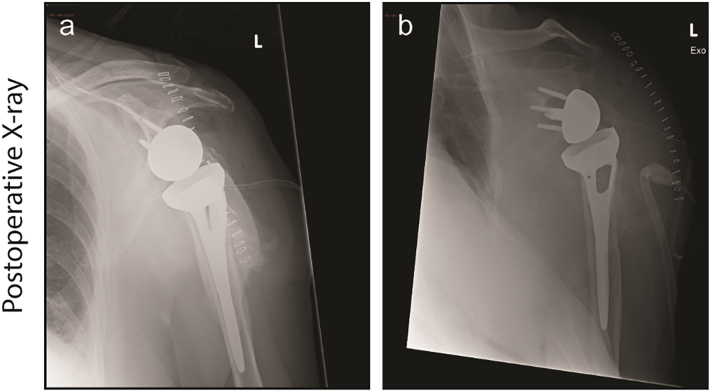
Control X-rays of the left shoulder one day after RSA: Free greater tuberculum, formation of callus and free osseous fragment laterally from the humeral shaft.
